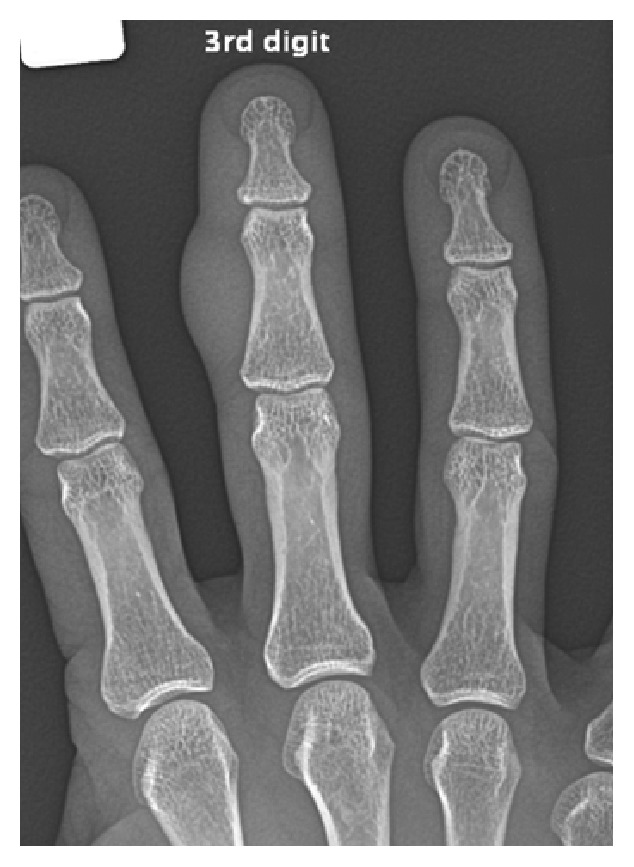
AP radiograph showing large fusiform soft tissue swelling of right middle finger at a level of the middle phalanx.
